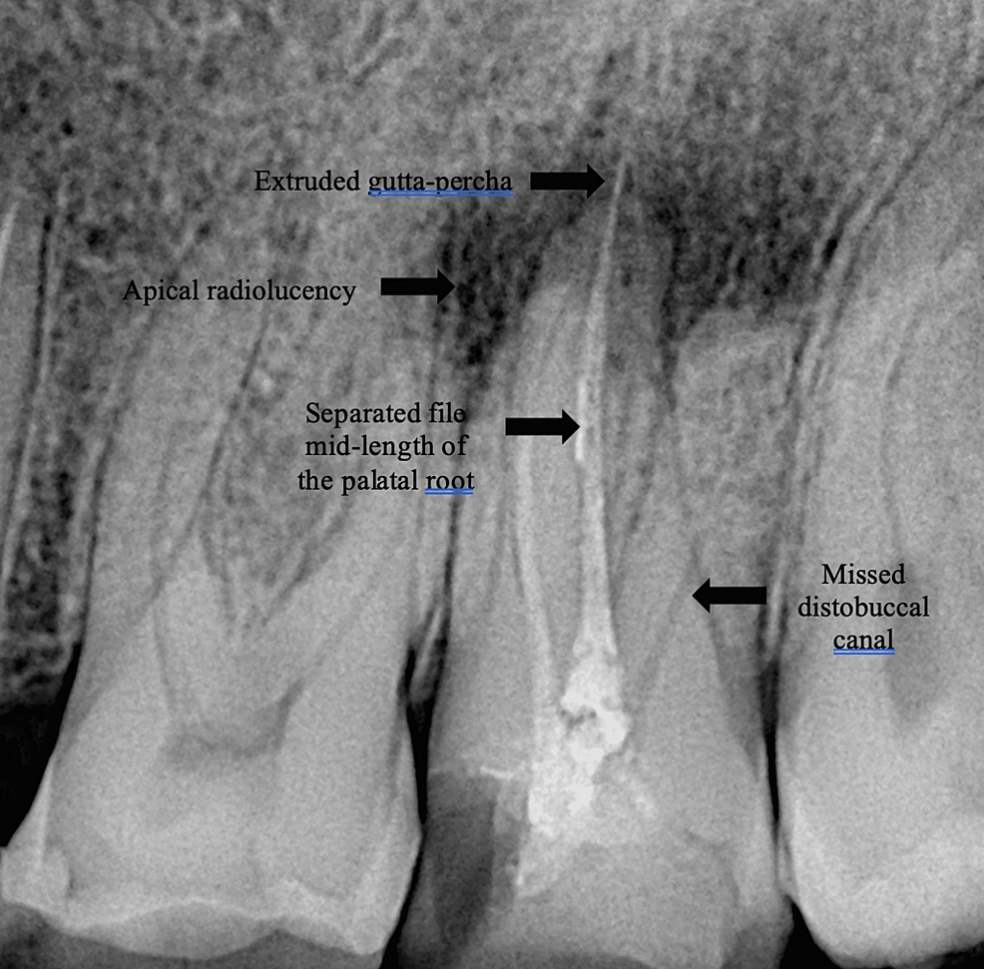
Preoperative radiograph of a maxillary left second molar with dental caries, substantial coronal destruction, missed canal, separated file, extruded gutta-percha, and periapical radiolucency.
